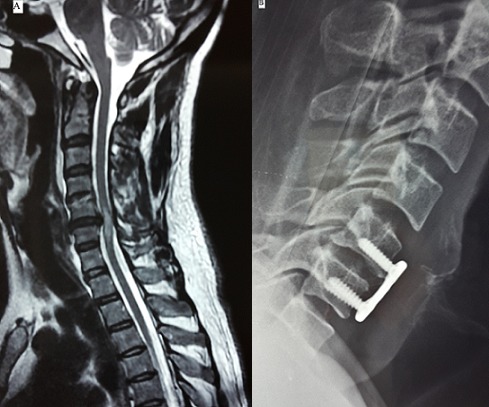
(A) IRM cervicale coupe sagittale en séquence pondérée T2 montrant une hernie discale en C5-C6 comprimant la moelle qui est siège d'une contusion centromédullaire; (B) radiographie cervicale de profil en postopératoire montrant la plaque cervicale et le greffon en C5-C6
